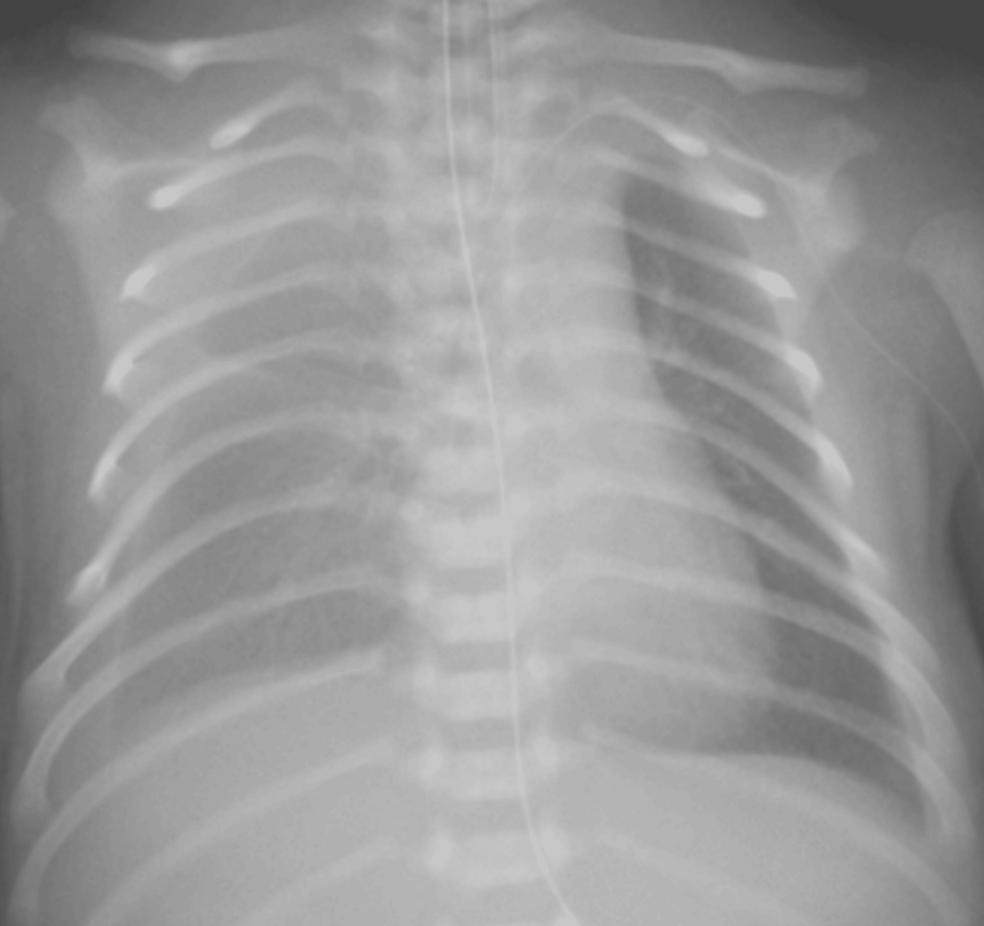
Chest radiograph on day one Increased right pleural effusion is seen.
